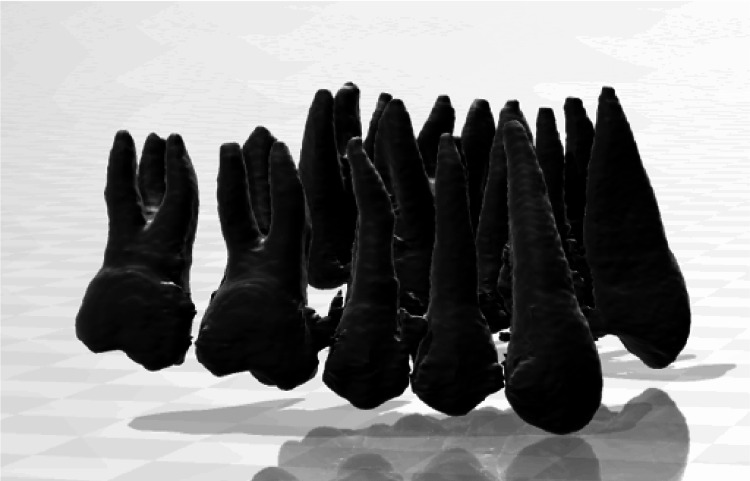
Cone-beam computed tomography image of the maxillary dental typodont model. This image visualizes the crown and roots.
